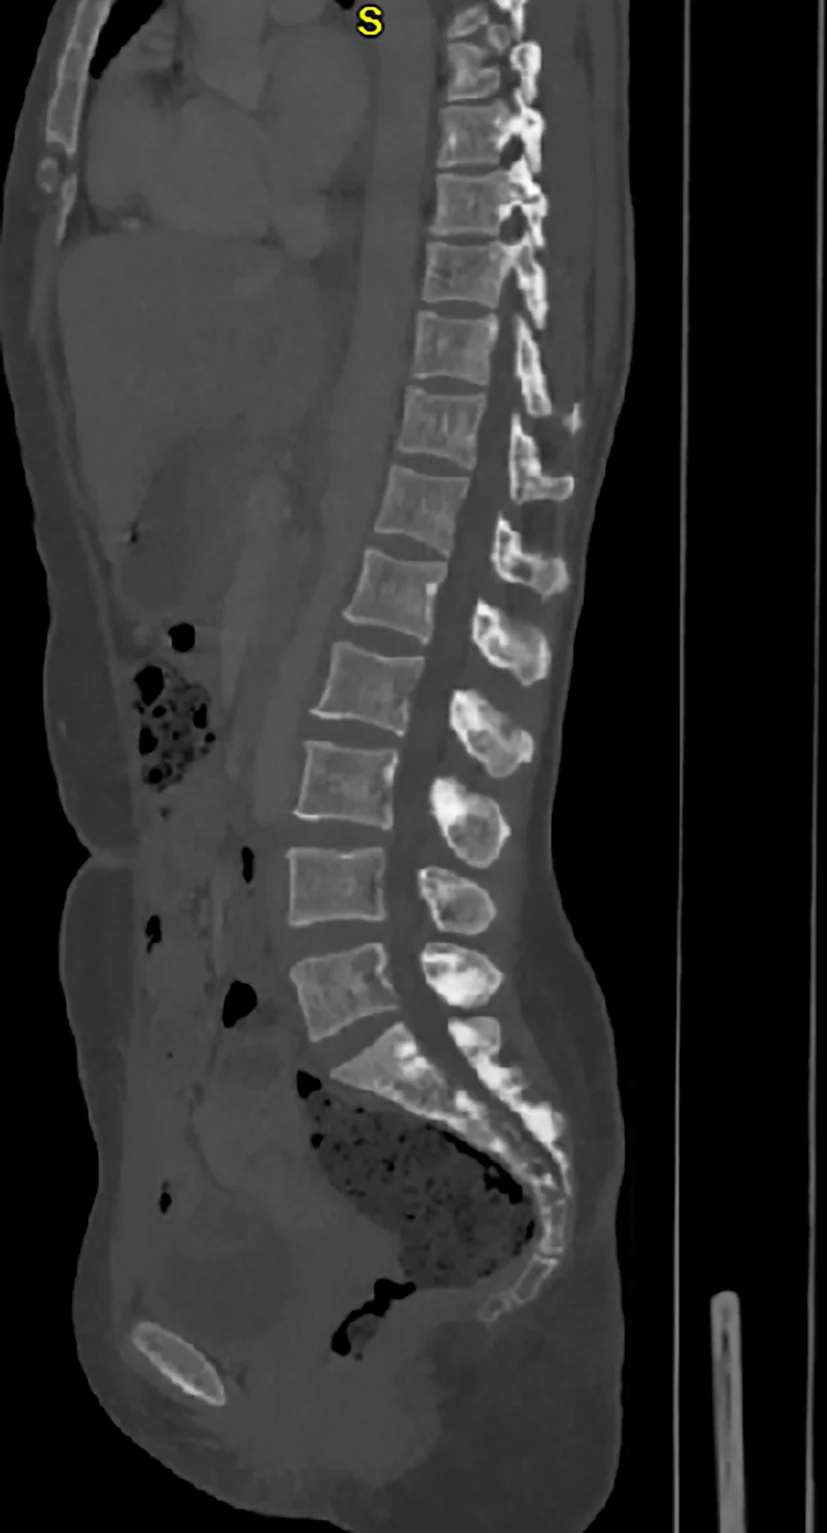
CT scan of the chest and spine showed that diffuse posterior element sclerosis is observed in the thoracic and lumbar spine.
